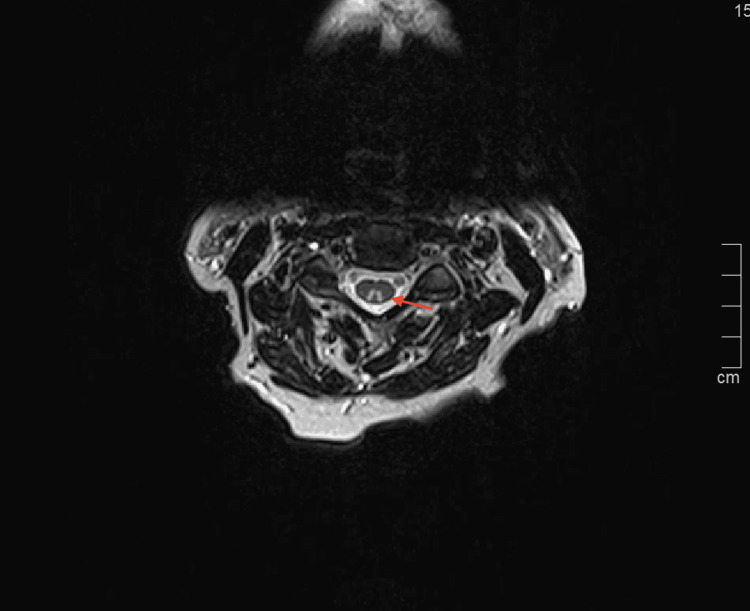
MRI cervical spine, axial T2 at the level of C3-4 showing dorsal column hyperintensity in a classic ‘inverted V’ pattern typical for subacute combined degeneration/copper myeloneuropathy (arrow).
